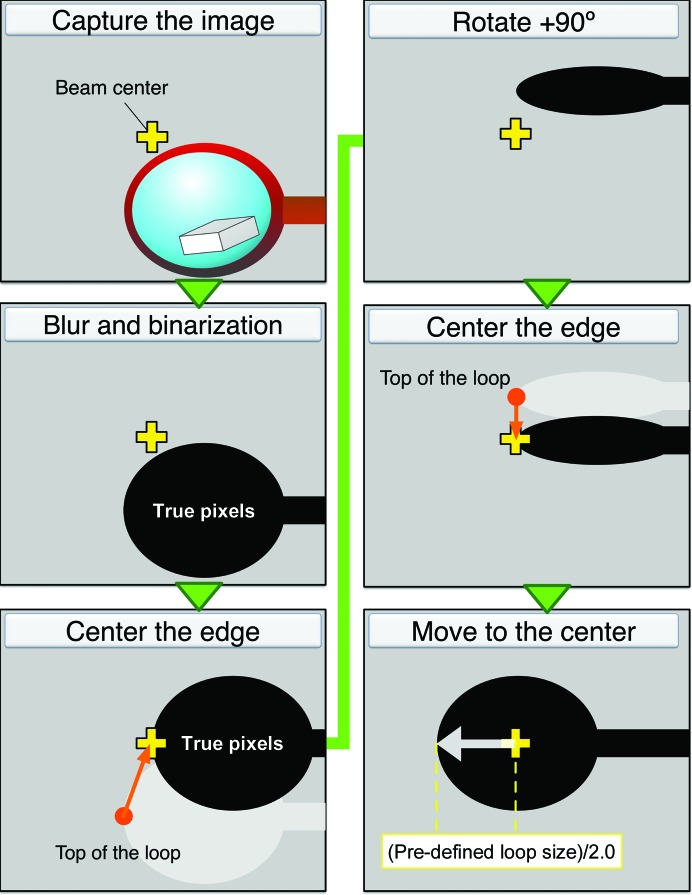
Loop centering by *INOCC*. After blurring and binarization of the captured image with *OpenCV* (Bradski, 2000 ▸), the program recognizes the left edge of the loop and translates it to the X-ray irradiation point at rotation angles of 0°, 45°, 90° and 135°. After convergence of the process, the face angle where the face of the loop is perpendicular to the X-rays is found. Finally, the program moves the loop to the left by a half-length of the user-defined loop size along the rotation axis.
